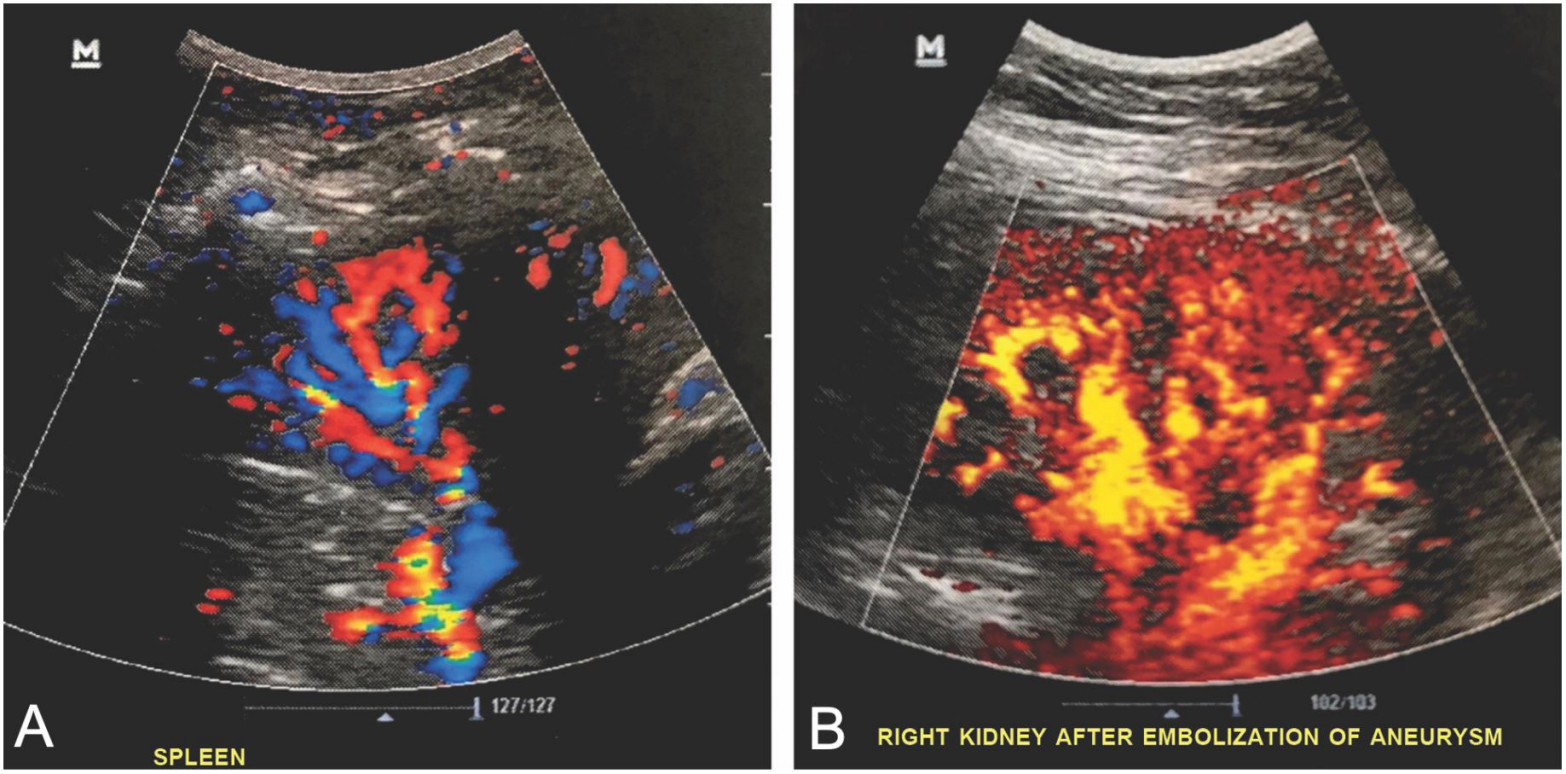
(A) Postoperative control Doppler ultrasonography images showing good
